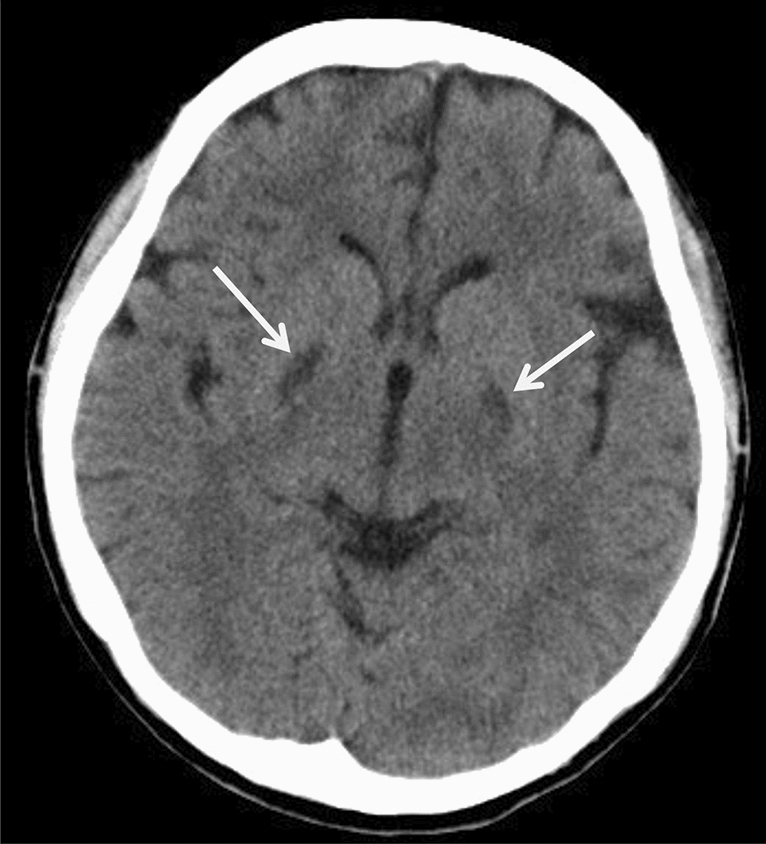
A 25-year-old, female patient with methylmalonic acidemia (MMA) and chronic renal failure. CT showed abnormally low density in the globi pallidi (arrows)
